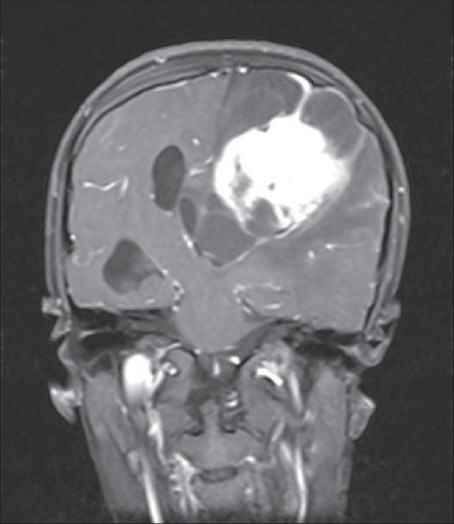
Coronal section of the contrast-enhanced magnetic resonance imaging image showing heterogenous enhancement of the tumor
